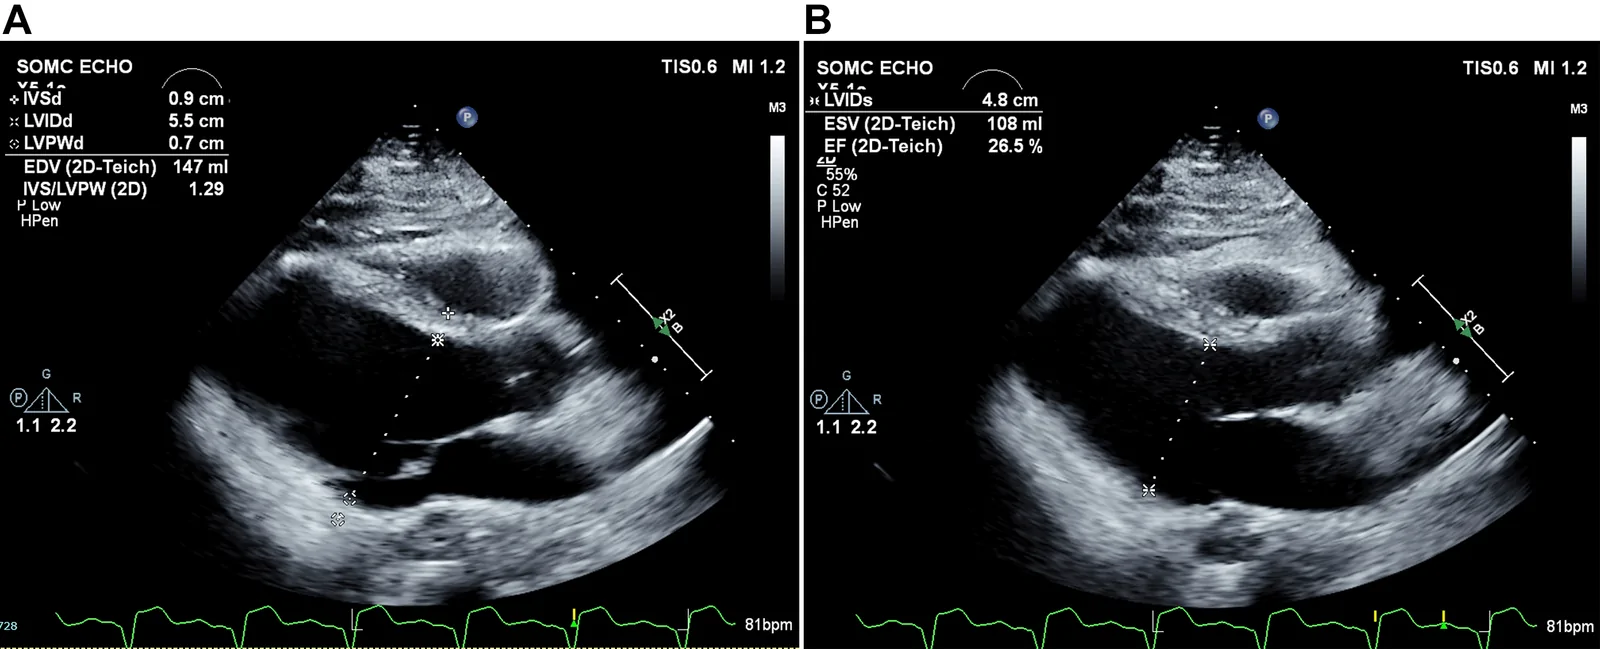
Baseline Transthoracic Echocardiogram in the Parasternal Long-Axis View Demonstrating Left Ventricular Ejection Fraction of 27% Before Initiation of Finerenone (A) End-diastole with left ventricular internal dimension at end-diastole (LVIDd) of 5.5 cm and (B) end-systole with left ventricular internal dimension at end-systole (LVIDs) of 4.8 cm. EDV = end-diastolic volume; EF = ejection fraction; ESV = end-systolic volume; IVSd = interventricular septum thickness at end-diastole; LVPWd = left ventricular posterior wall thickness at end-diastole.
